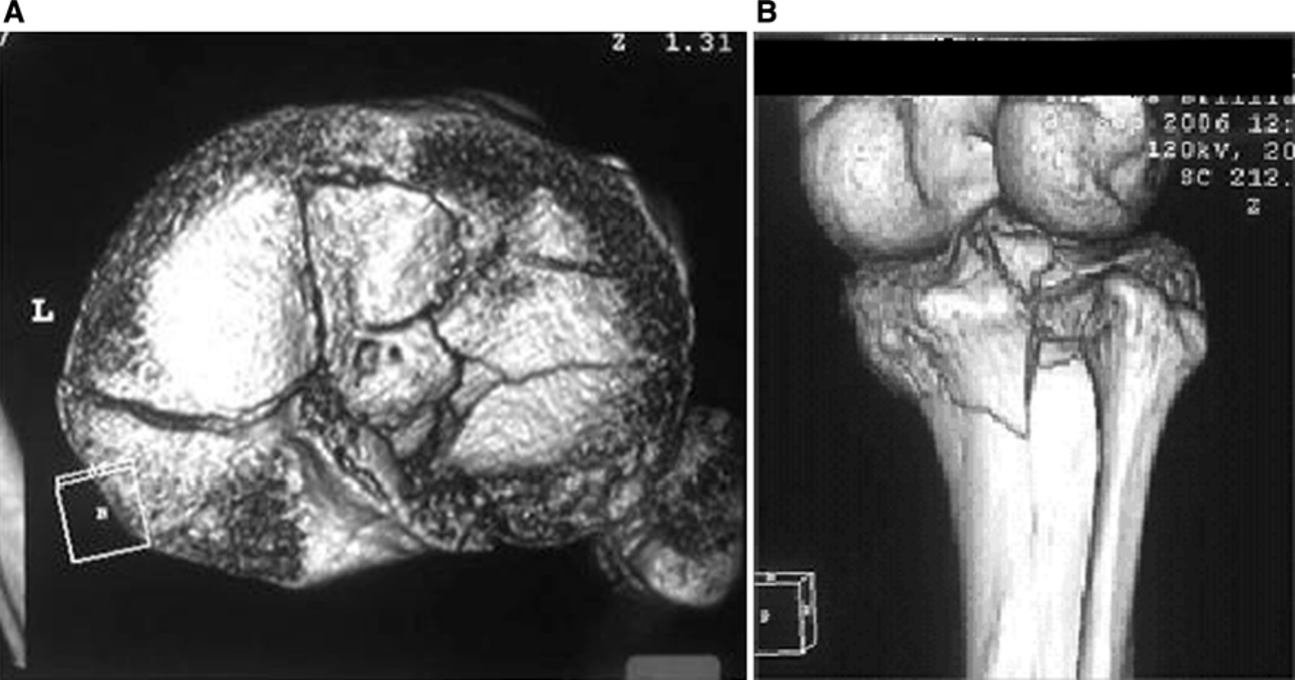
a Schatzker type V fracture, CT 3D reconstruction. b Schatzker type V fracture, CT 3D reconstruction
